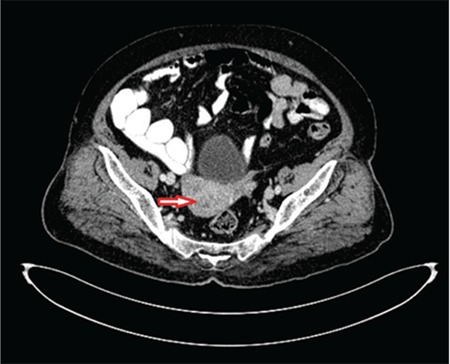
Abdominal computed tomography showing heterogeneous appearance in the endometrial cavity
